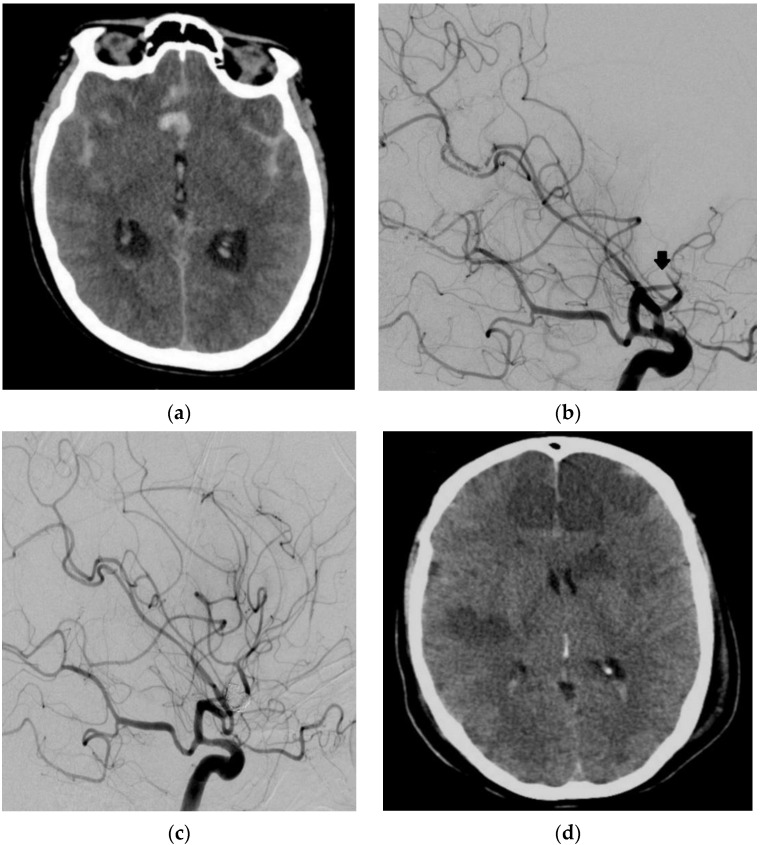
Diagnostic imaging and EVT in a 39-year-old female patient with an aSAH due to a ruptured anterior communicating artery (AcomA) aneurysm. Cranial CT showed an aSAH (a). DSA with a contrast-medium injection of the left ICA artery (lateral view 45°) showed an embolic occlusion of the superior trunk of the left MCA (arrow) and ACA (b) with the dissolution of the thrombus after infusion of rtPA (c). Cranial CT showed massive ischemic infarctions of both ICA and MCA supply territories (d).
